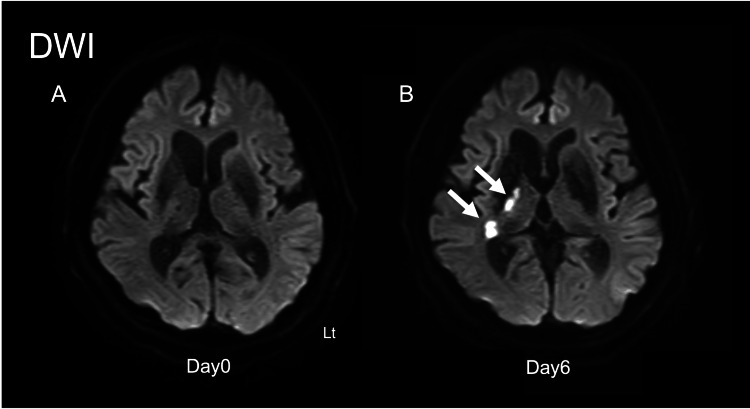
Head MRI images DWI: diffusion-weighted imaging; PLIC: posterior limb of the internal capsule; LGN: lateral geniculate nucleus (A) DWI horizontal section on day 0 of onset. (B) DWI horizontal section on day 6 of onset. The white arrows indicate high signal intensity in the right PLIC and LGN, a finding not observed on day 0
